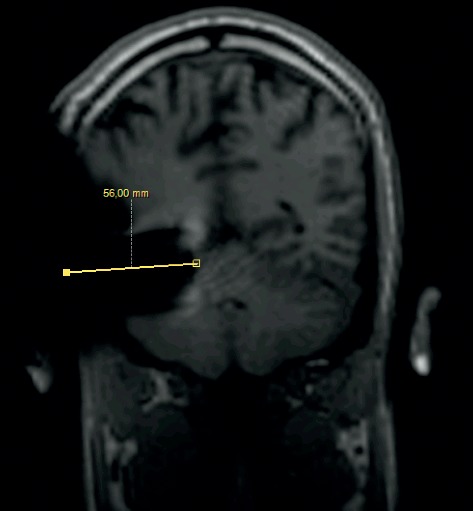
MRI survey pattern of the coronal plain of a 1.5 T scanning of an Oticon ZTI Implant.
